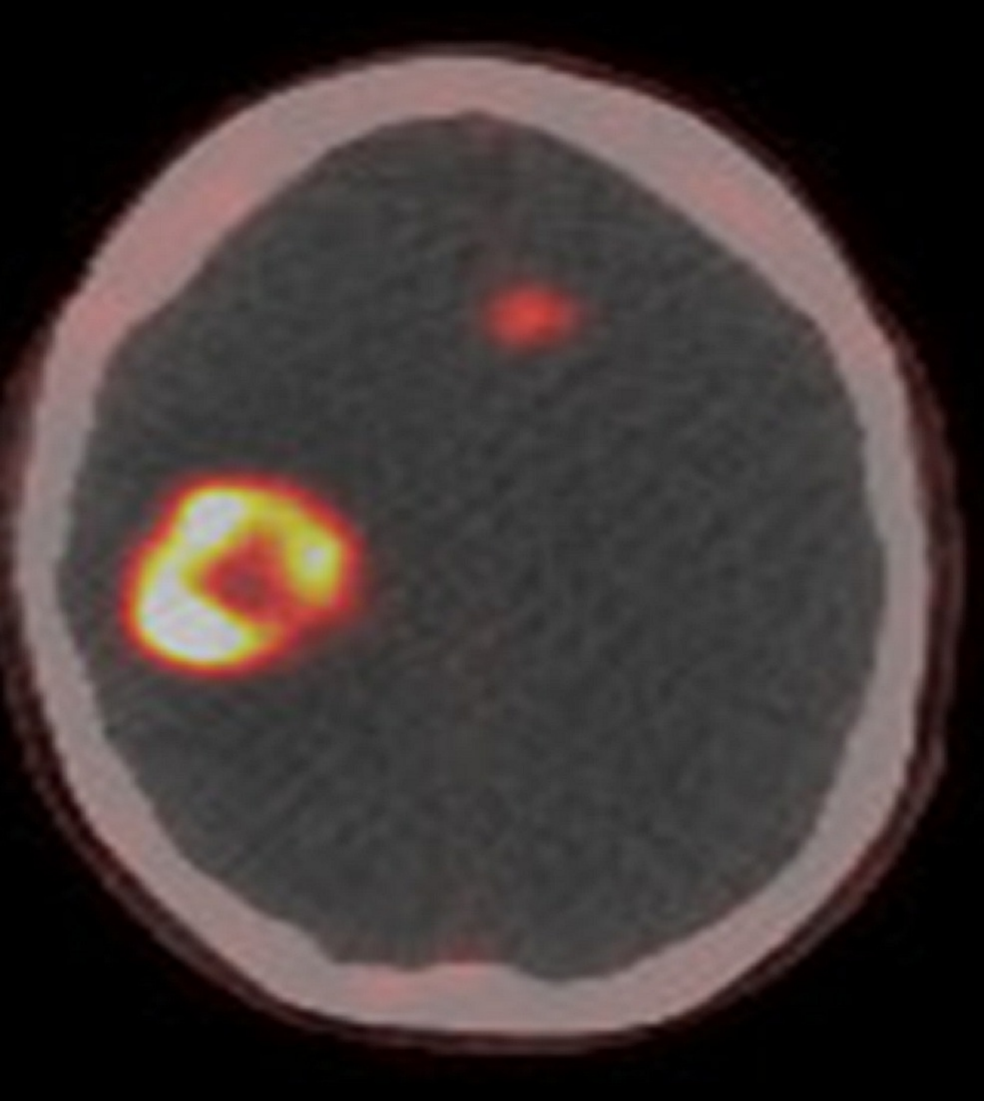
68Gallium DOTATATE positron emission tomography−computed tomography imaging (axial) of the brain shows a right parietal lesion and left frontal lesion.
